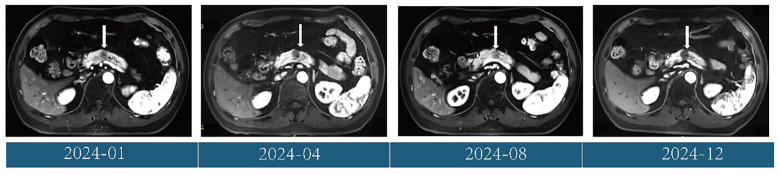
Changes in the pancreatic metastases (white arrow) during treatment, shown on MRI (T1 weighted image).
